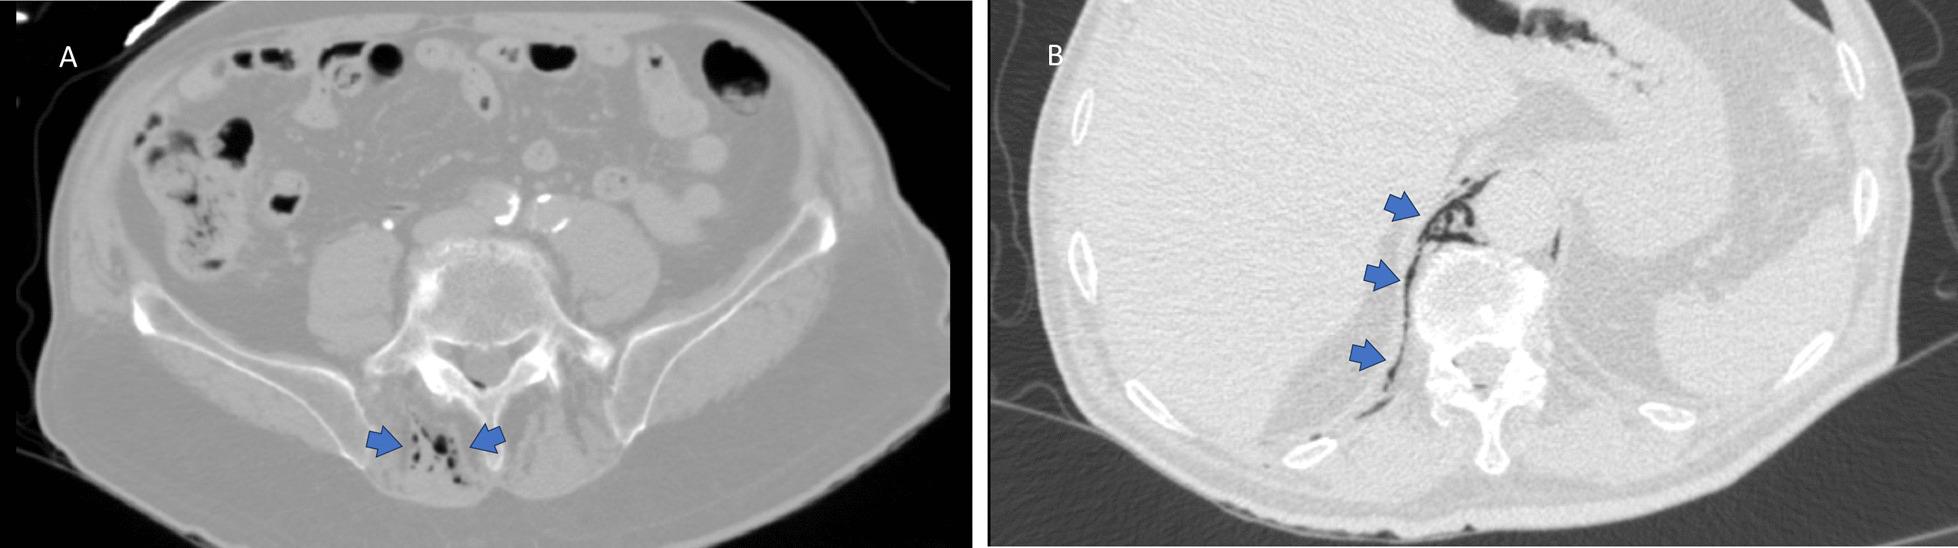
CT Scan of the abdomen A Free air (arrows) present in the subcutaneous tissue at the puncture site and in the epidural space; B Air (arrows) present in the paravertebral soft tissue and around the abdominal aorta
